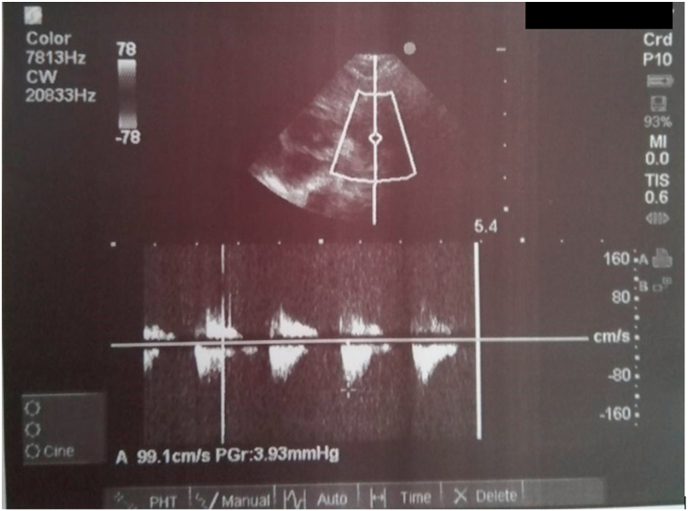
The result of brain ultrasonography, showed choroid plexus 2.7 (mm).
